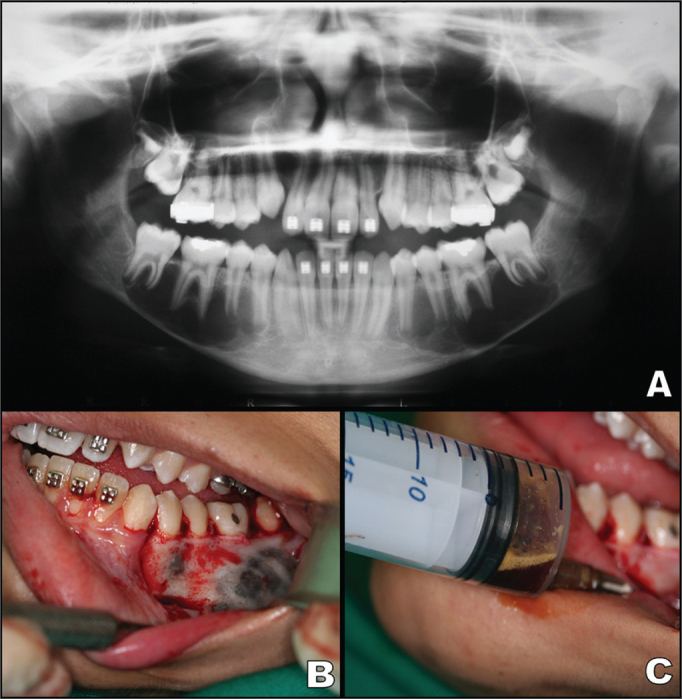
A: Panoramic radiograph showing a bilateral multilocular lesion with scalloping along the roots of teeth in the posterior area of the mandible. B: Intraoral view after making a mucoperiosteal flap to show bone expansion with hemorrhage. C: Aspiration biopsy showing serous-bloody fluid.
